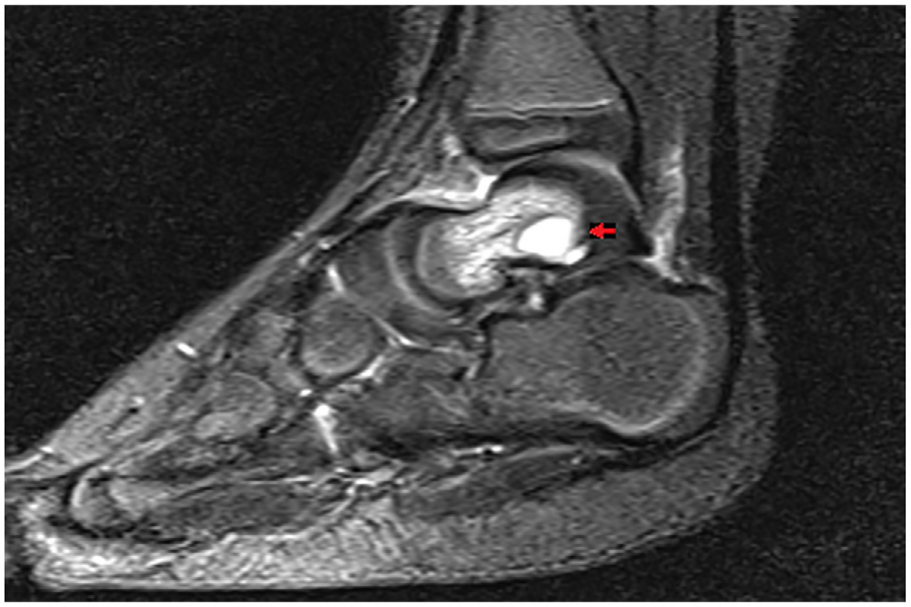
MRI STIR sequence imaging of the right foot, showing abnormal focal and diffuse hyper-intense signal changes in the posterior aspect of the talus.
